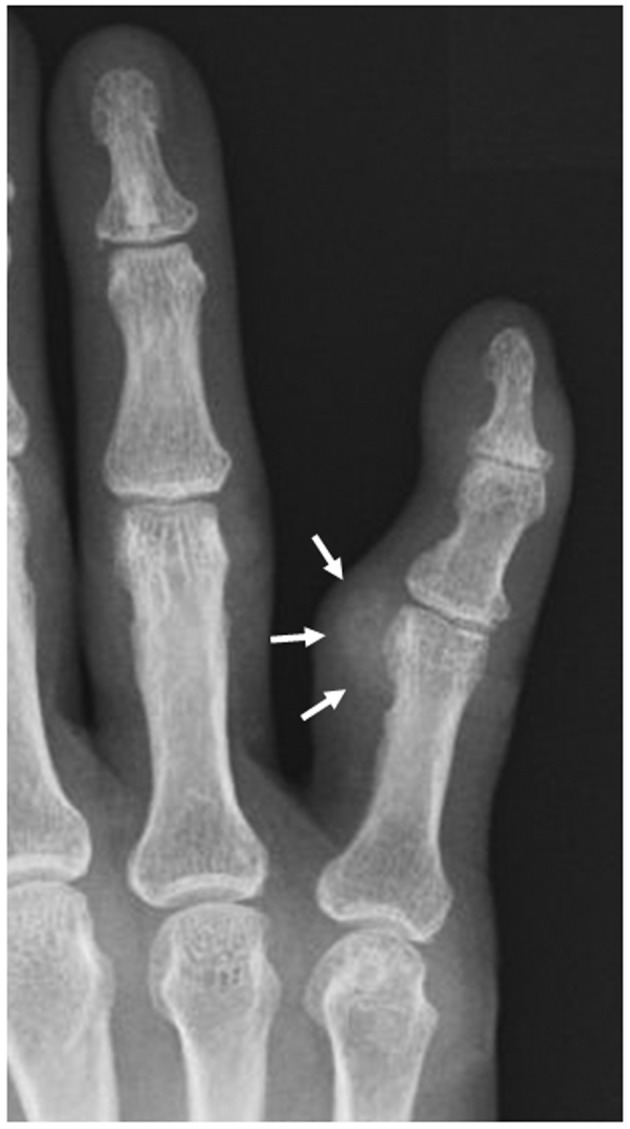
Plain radiograph reveals a faintly calcified soft tissue mass (indicated by the white arrows) without bone involvement.
